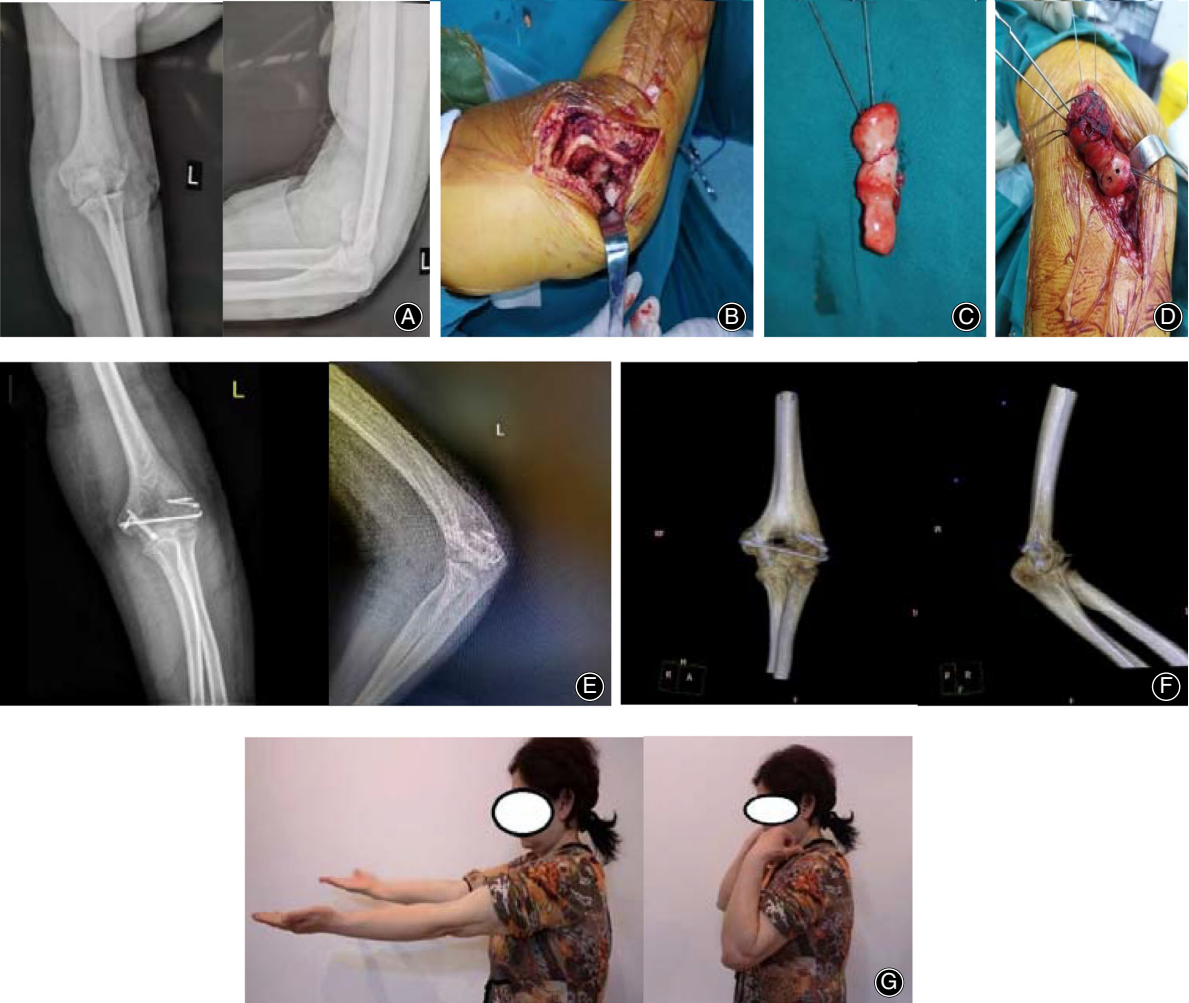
(A) X‐ray imagines showed comminuted fracture of capitellum and trochlea. (B) Trochlear fracture was exposed by additional medial incision. (C) The free fragments were taken out of the body. (D) The fracture fragments were fixed as a whole with Kirschner wires and suture. (E) X‐ray radiographs of the elbow after operation shows three headless screws (DART‐FIRE headless screw produced by WRIGHT company, Memphis, USA), two 1.0mm diameter Kirschner wires and suture were used to fix the fracture. (F) CT with three‐dimensional imagines showed anatomical reduction of fracture and satisfactory implants position. (G) The images of elbow 1 year after operation, the extension was 5° and flexion was 130°. The patient had a good functional elbow outcome with a MEPS was 85.
